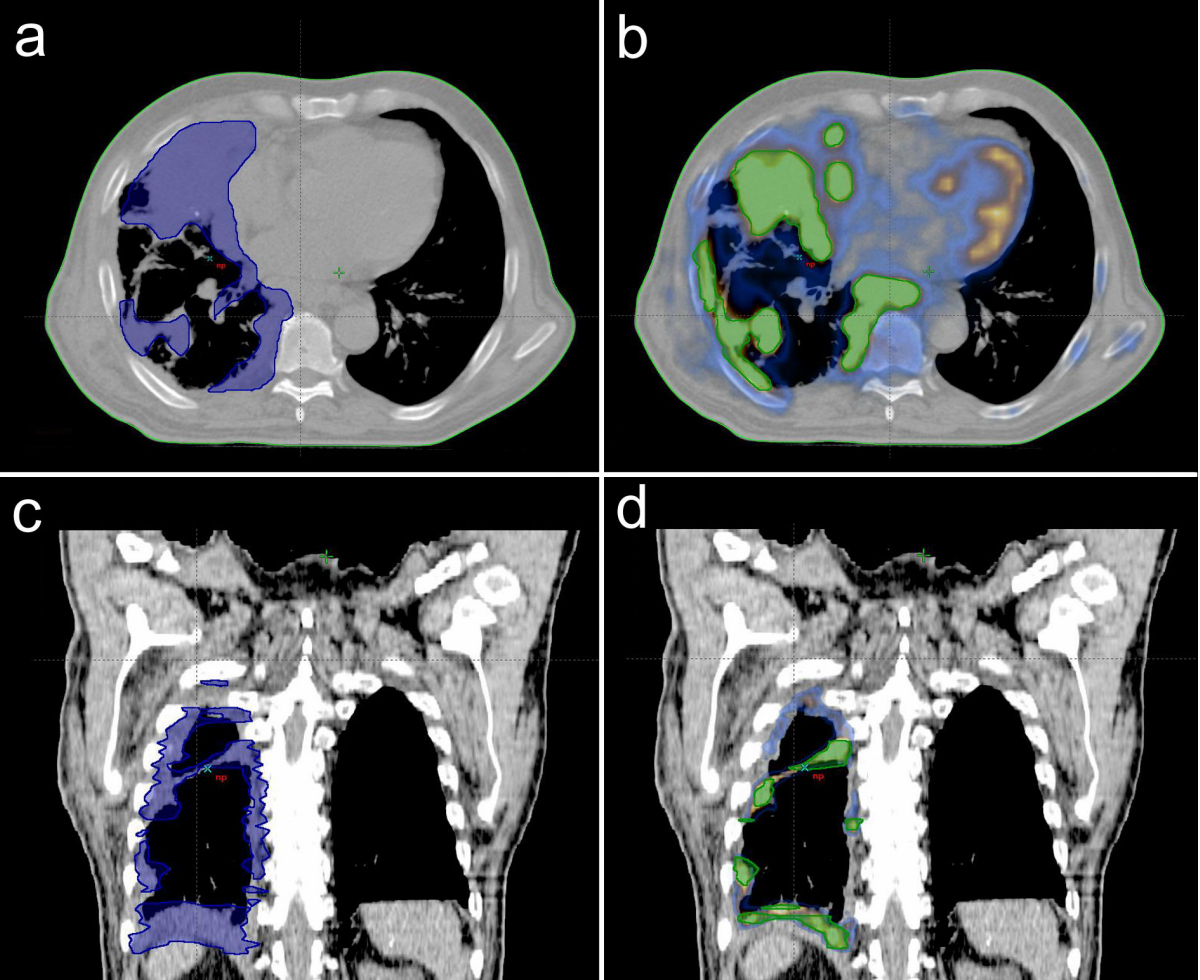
Representative image of a patient with CT- and PET- CT based GTV delineations; (a) axial CT (b) axial PET-CT, (c) coronal CT, (d) coronal PET-CT. Abbreviations*: GTV = gross tumor volume; CT = computed tomography; PET-CT = positron emission tomography-computed tomography.
